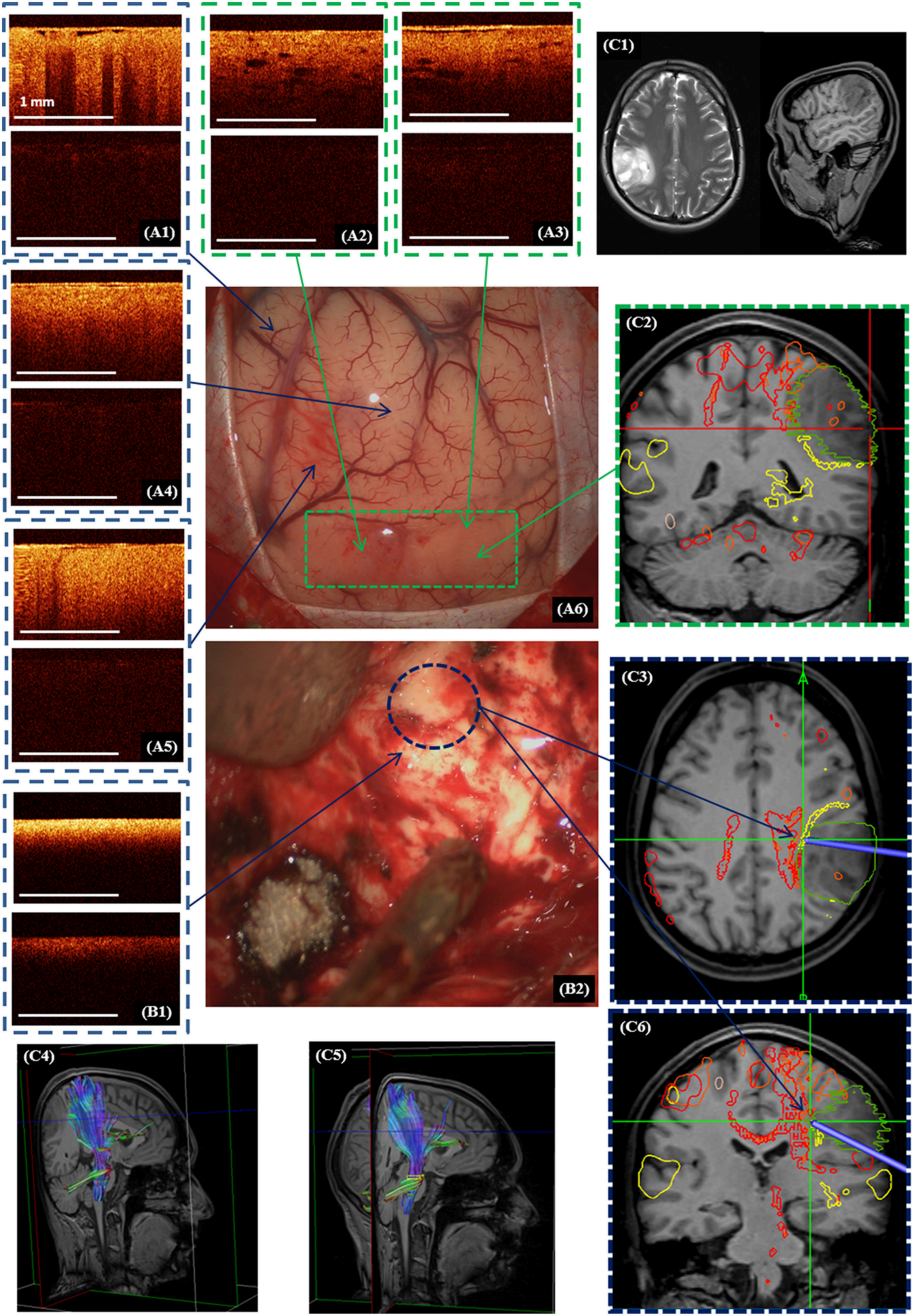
Intraoperative CP OCT images in a patient with diffuse astrocytoma (Grade II): of the cortex (A1) with well-defined (A2,A3; A6—green dotted line) and invisible (A4,A5) tumor invasion, white matter (B1) close to the right corticospinal tract (B2—blue color dotted line; C3,C4—marked by red color); the scanning areas of corresponding tissue types in the surgical field (A6,B2) are marked with green (A6) and, blue (B2) dotted lines and interrelated with the neuronavigation data, where the tumor is marked with a green line and the corticospinal tract with a red line (C2,C3,C6). Preoperative MRI in T1 and T2 (C1) and the corticospinal tract reconstruction based on the DTI before (C4) and after (C5) operation. (A1–A5,B1)—CP OCT images in co-polarization (upper image) and cross-polarization (lower image).
